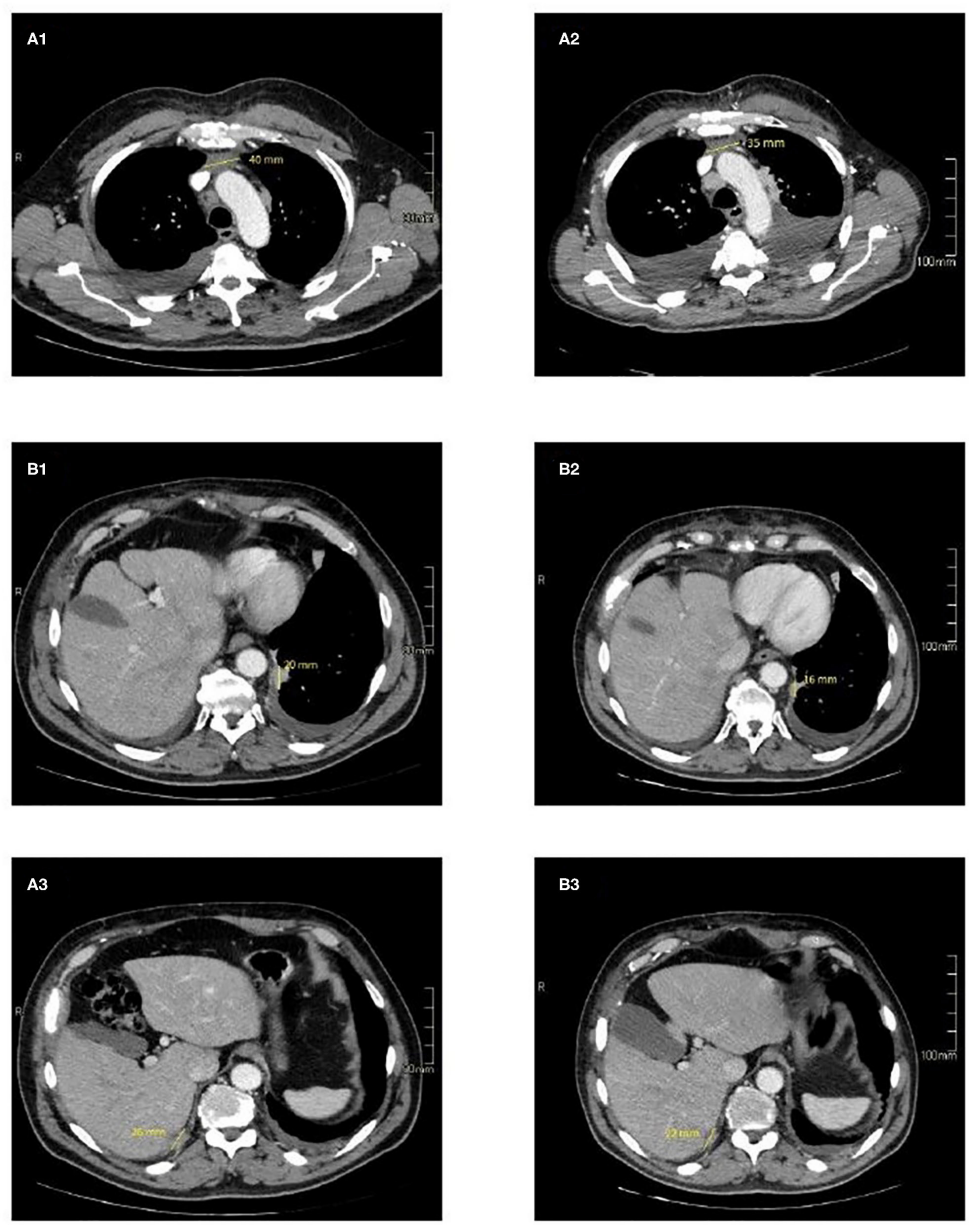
CT imaging of selected target lesions at baseline (A) and during treatment (B). Representative axial CT images of patient shows a slight reduction in size of the primitive tumour, 35 mm (B1) vs. 40 mm (A1) (blue arrows) and pleural localizations on the left side 16 mm (B2) vs. 20 mm (A2) (yellow arrows), and right side 22 mm (B3) vs. 26 mm (A3) (red arrows).
